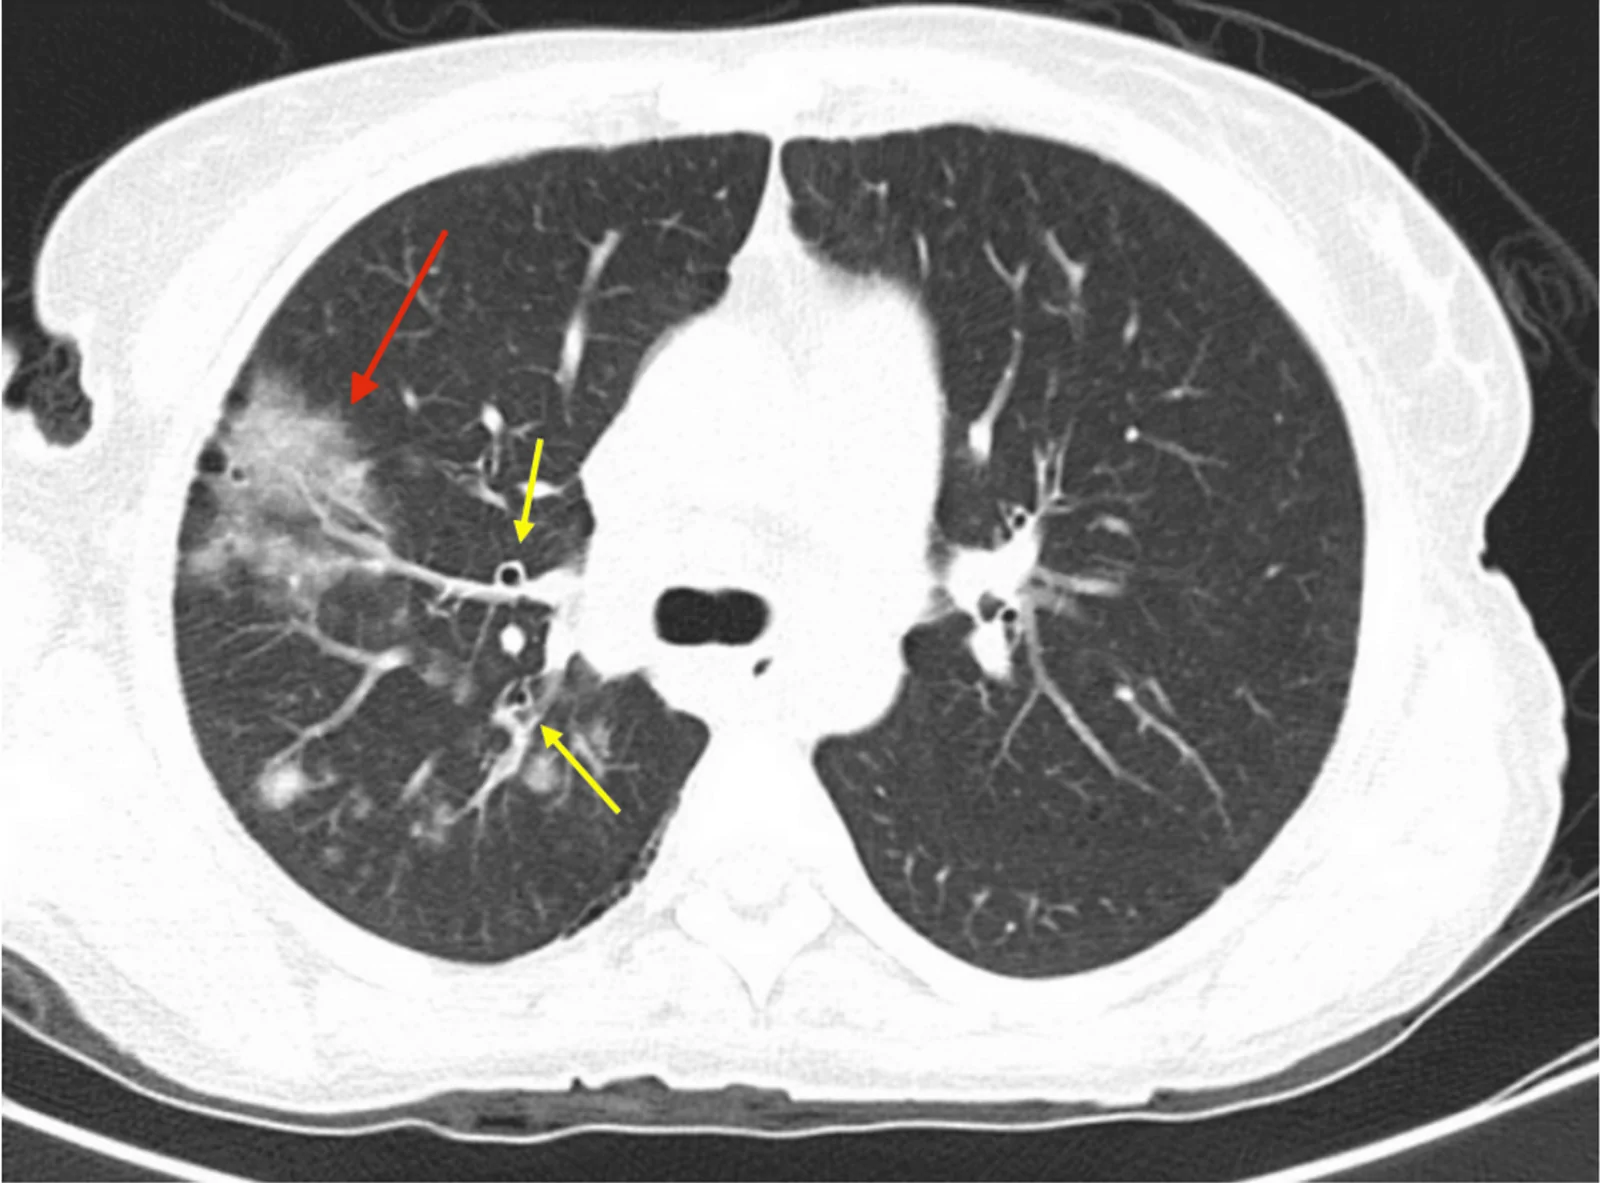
Chest non-contrast CT scan demonstrating ground-glass opacities (red arrow) and multiple cavitary components (yellow arrows) in the right lung consistent with pneumonia During one of her many hospital admissions after being diagnosed with AIDS, the patient was found to have pneumonia. Her imaging, which demonstrates ground-glass opacities (red arrow) and cavitary components (yellow arrows), can be seen above. The diagnosis of pulmonary cryptococcosis was also confirmed on laboratory testing. Pneumonia is common in people with severely suppressed immune systems due to the body's inability to fight off lung infections. This illness was only one of the many sequelae this patient faced as a result of barriers to continuous HIV treatment. CT: computed tomography; AIDS: acquired immunodeficiency syndrome; HIV: human immunodeficiency virus
